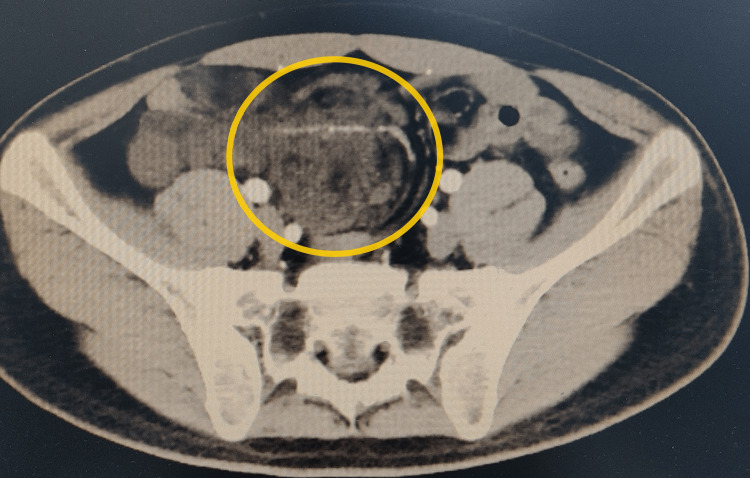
Axial CT scan of the abdomen The yellow circle indicates the mesenteric mass. The abdominal CT scan indicates a mass in the mesentery of the small intestine, measuring approximately 114*62*127mm, showing visible mesenteric blood vessels and slightly enlarged lymph nodes.
